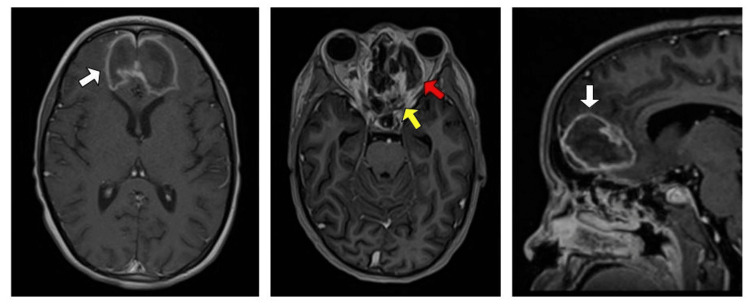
MRI of the skull showing signs of invasive fungal sinusitis with extension to the central nervous system and orbital cavities Note the mucosal thickening in the left maxillary and sphenoid sinuses (yellow arrow); extensive heterogeneous hypovascular area involving the frontal lobe, cribriform plate, and olfactory bulb, infiltrating the medial portions of the adjacent frontal lobes and anterior portion of the corpus callosum (white arrows); areas of necrosis within the orbital cavities, with infiltration of the ocular muscles and proptosis (red arrow).
